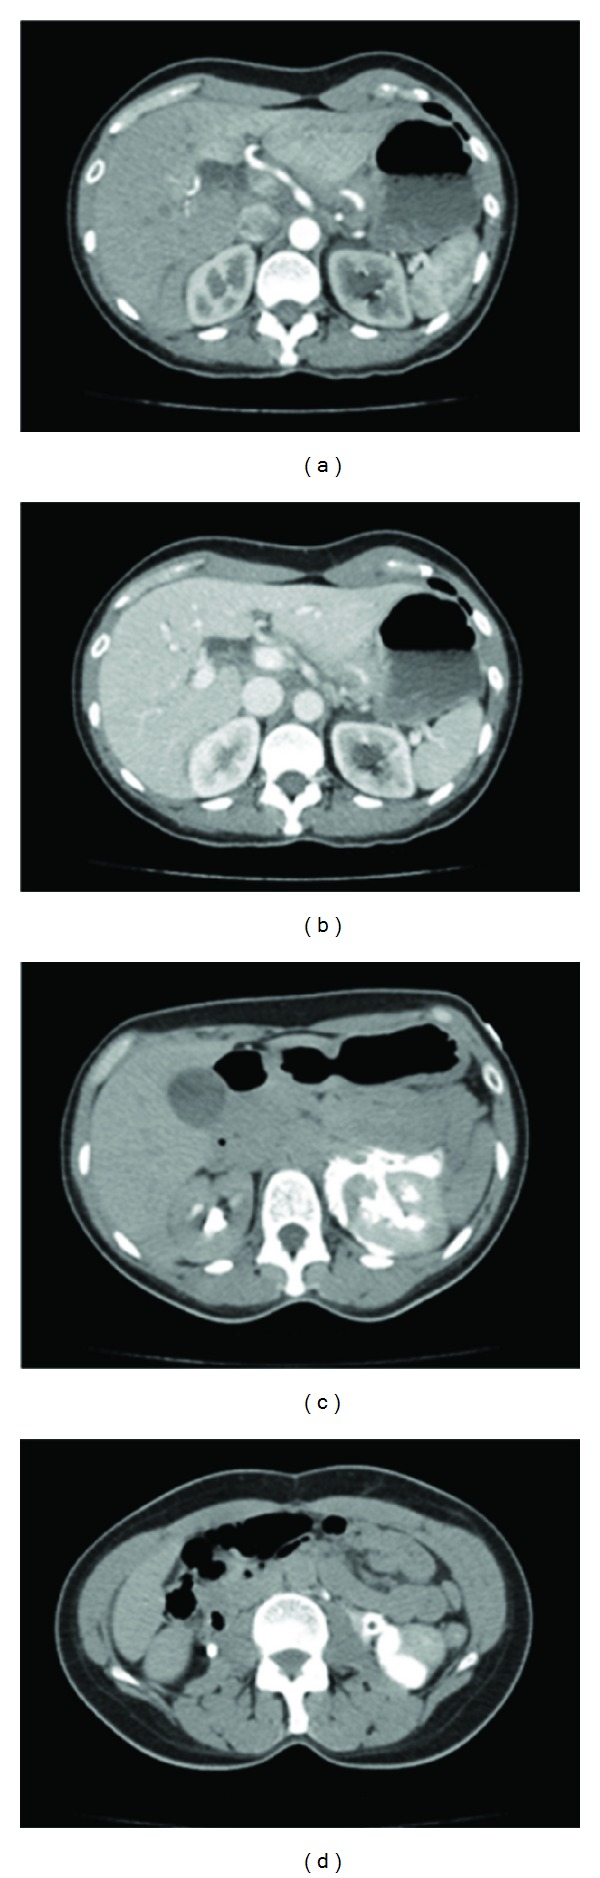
(a) CT arterial phase, (b) CT venous phase, (c) and (d) CT with delayed venous phase showing renal extravasation of IV contrast.
